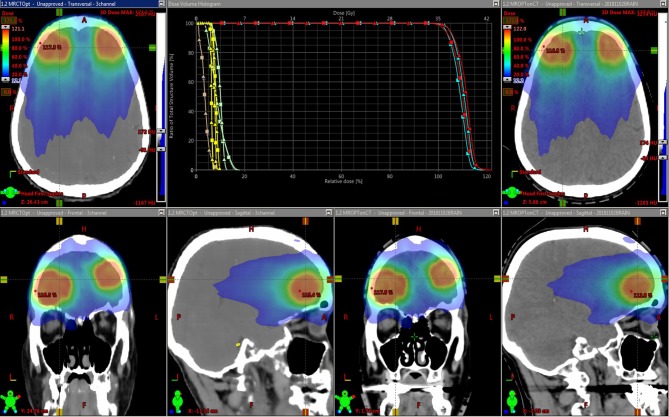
Dose distributions for intensity modulated treatment plans for two targets. The original plan was optimized using the MRCT-derived density grid (left), and the resulting beam fluences were used to recalculate doses on the CT-derived density grid (right). Dose volume histograms (DVHs) for the Brainstem (yellow), Optic chiasm (brown), eyes (green), and two targets (light and dark blue) are shown. Squares represent MRCT plan DVH curves, and triangles come from recalculated plans using CT.
